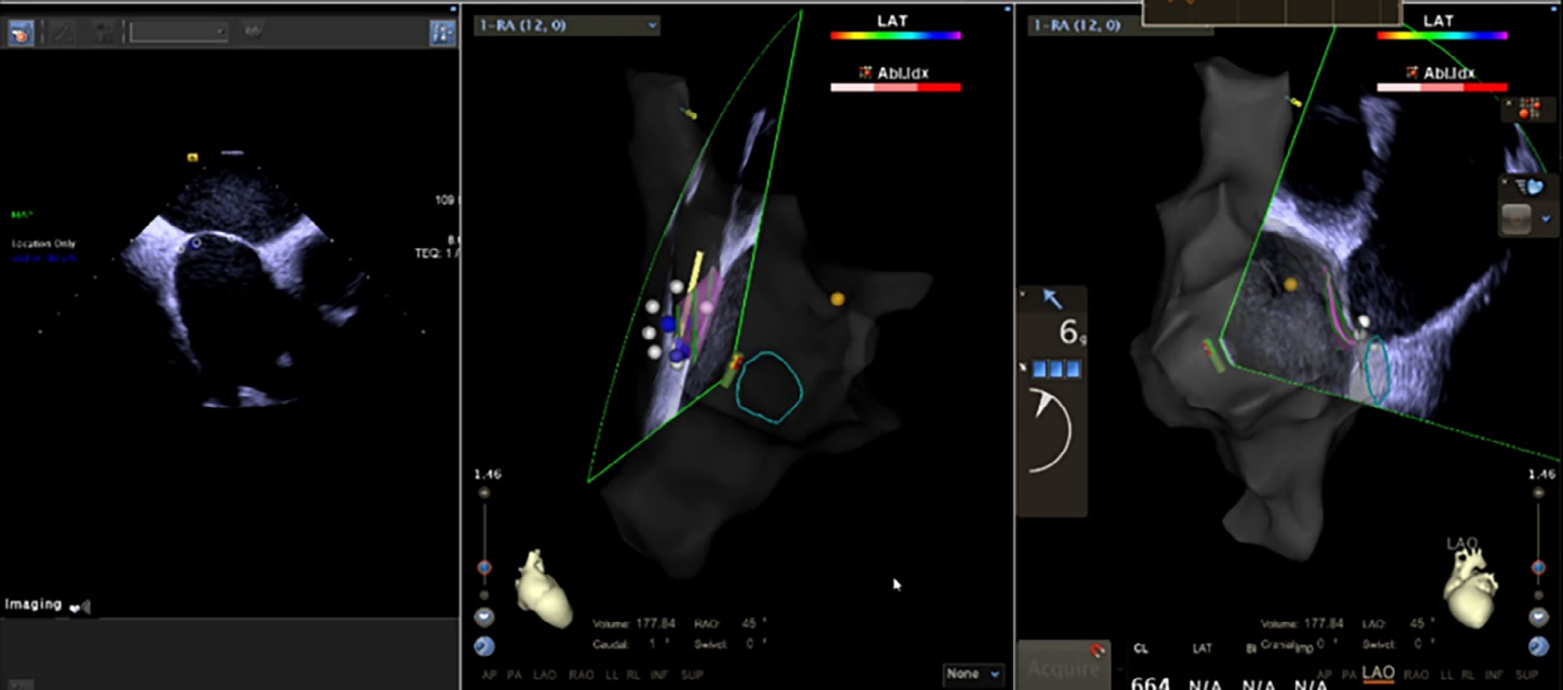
Intracardiac echocardiography (ICE) was performed to confirm the position of the foramen ovale. An ablation catheter and electroanatomical mapping systems (EAM) were used to map the anatomy of the foramen ovale. The position of the foramen ovale was confirmed from two readings. (pink area, foramen ovale as mapped by ICE; yellow point, bundle of His; white point, area of low voltage identified by voltage mapping; green area/circle, coronary sinus)
